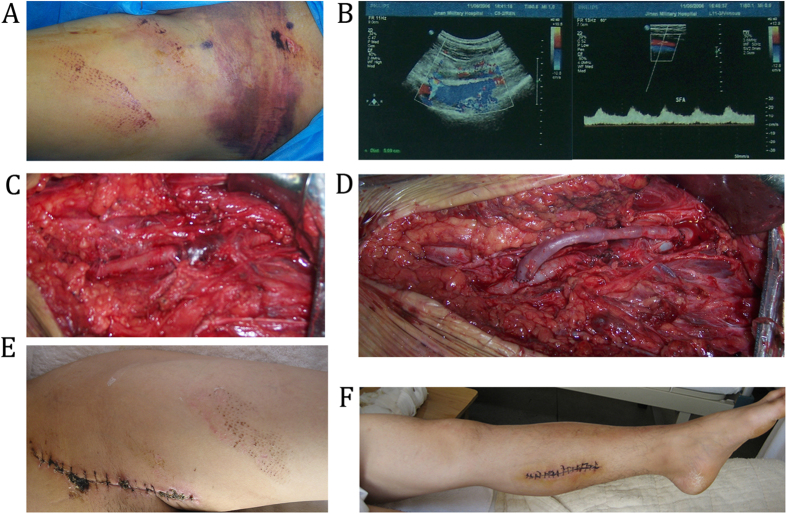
A 32-year-old man presented to the emergency department after sustaining a blunt injury to the root of his left thigh in a traffic accident. (A) Preoperative detection found that Blunt injury extending from the common iliac artery to the femoral artery trunk. (B) A color ultrasound was used to examine the vascular injury. (C,D) Reversed saphenous vein grafting (RSVG) to bypass the section containing the thrombus. (E,F) the patient recovered quickly and he was discharged from hospital after achieving a Fugl-Meyer upper-extremity score of 96 points.
